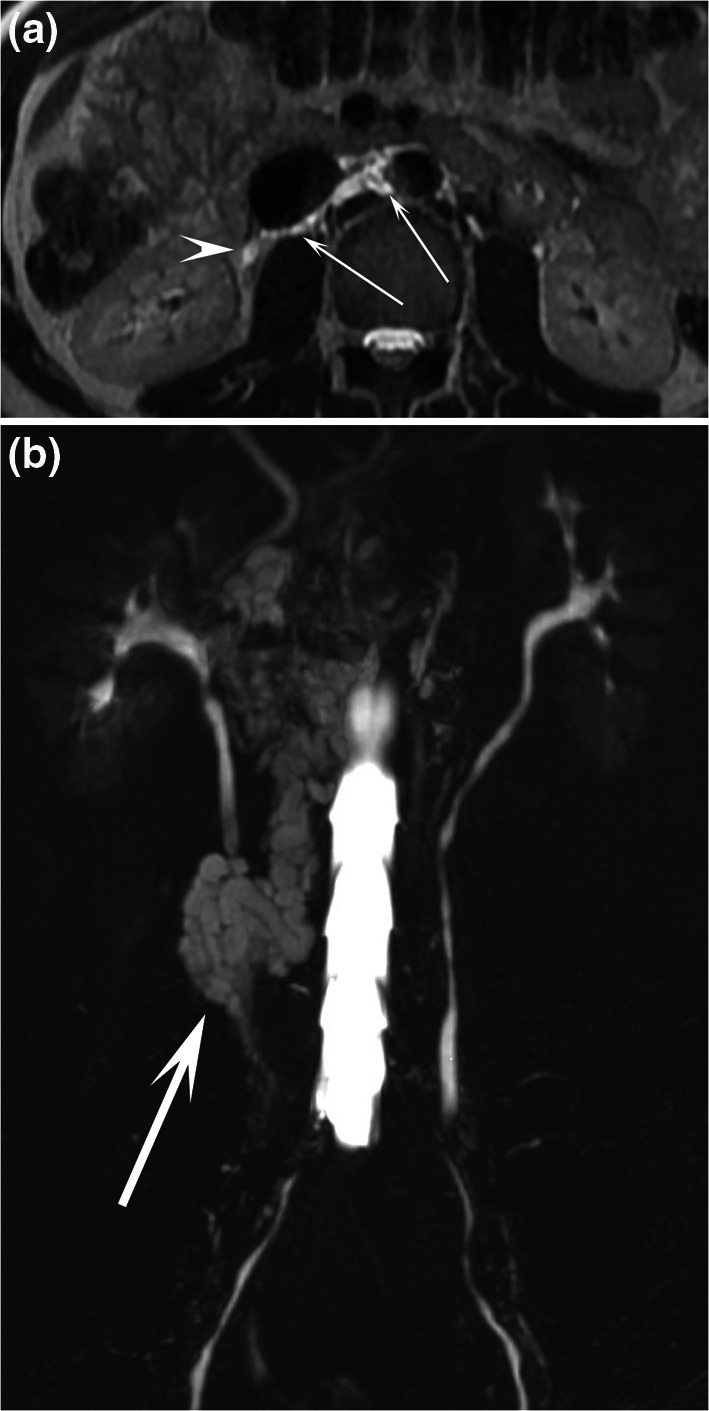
35-year-old male patient with chyluria. Axial T2-weighted MR image a demonstrated dilatation of the retroperitoneal lymphatic vessels prevailing on the right side (arrows) and in touch with the right ureter (arrowhead). Coronal MR lymphography with MIP reconstruction (b) demonstrated a channel type lymphatic malformation (arrows) in contact with the right renal pelvis and right ureter
